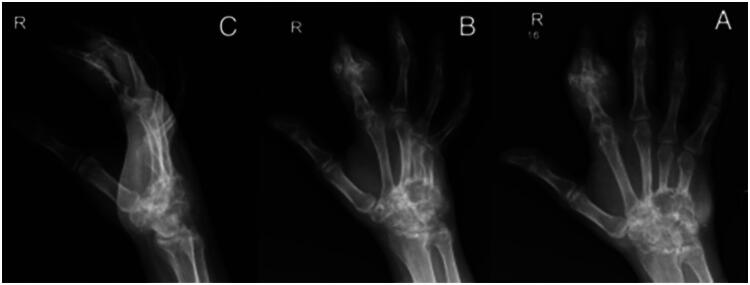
Left hand radiograph: (A) posteroanterior, (B) oblique, and (C) Lateral view. Shows significant left index finger soft tissue involvement with erosive changes and features of osteomyelitis.
